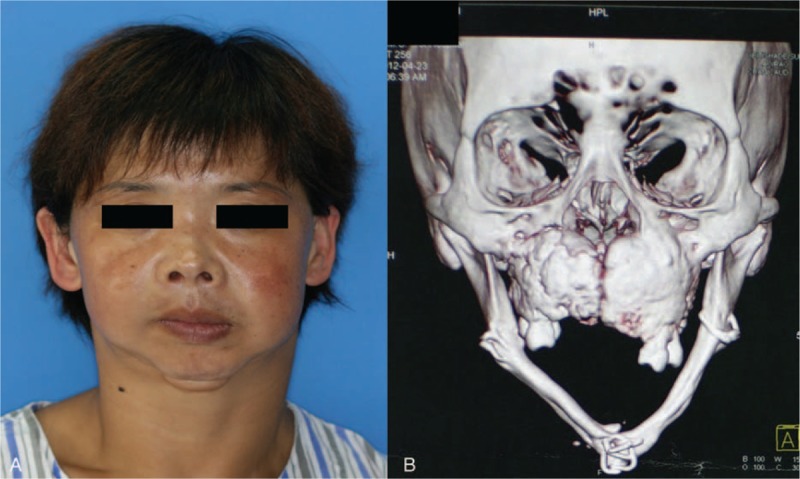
(A) The adolescent patient's aunt who had received surgery (nonvascularized iliac bone reconstruction) 30 years ago now complaint of anterior maxillary mass with chronic infection. (B) Reconstructed computed tomography image of familial gigantiform cementoma appearance.
